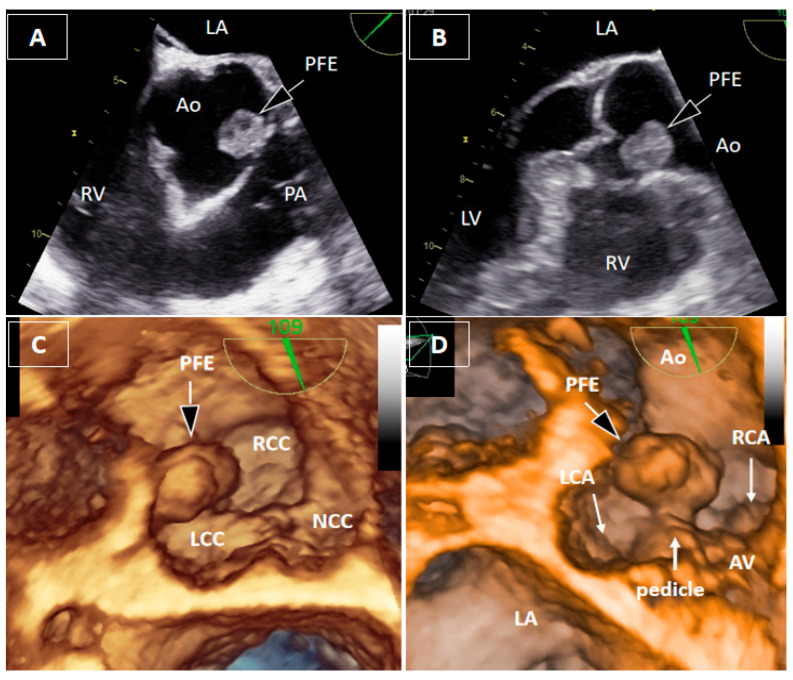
Transesophageal echocardiography. Two-dimensional transesophageal echocardiography (2DTEE) localized the mass to the region of the right coronary sinus, between the left and right coronary cusps, without precise identification of the attachment site (A,B). Three-dimensional transesophageal echocardiography (3DTEE) provided detailed anatomical definition, clearly identifying a short pedicle originating from the aortic wall between the bases of the left and right coronary cusps (C,D). The mass measured 17 × 11 × 8 mm, with a pedicle length of approximately 4 mm, and demonstrated marked mobility toward the right coronary artery ostium during the cardiac cycle. Measurements were obtained from the 3DTEE.
